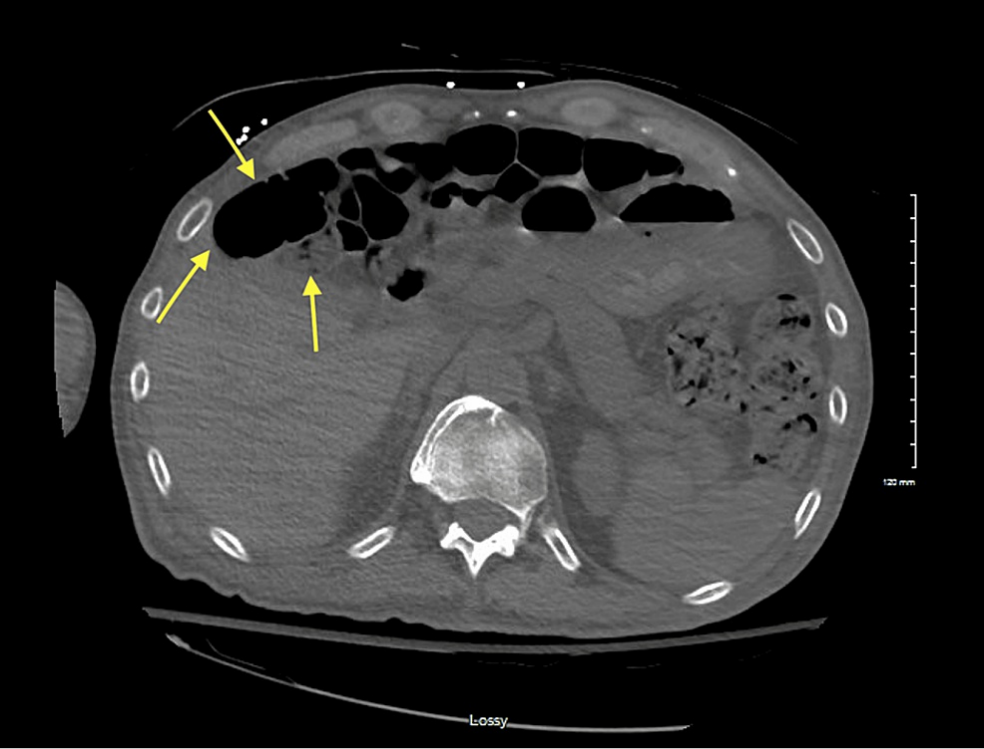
Abdominal CT one year prior to presentation Image showing CT scan of the abdomen with the bowel loops between the abdominal wall and the liver. The arrows pointing at the bowel loops CT: computed tomography
